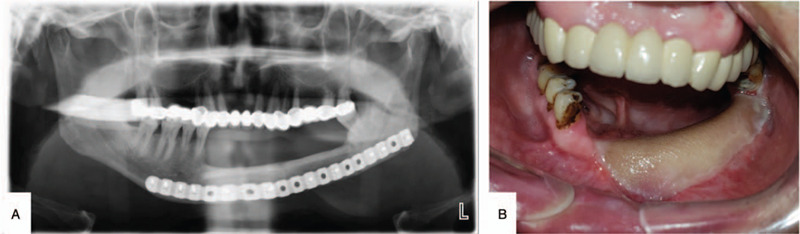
At 8 mo postoperatively, the follow-up panoramic radiograph indicated good bone healing. (A) The graft bone had incorporated well with the residual mandible, and the mandible contour was restored well. (B) The skin paddle in the mouth was in good condition and functioned well.
